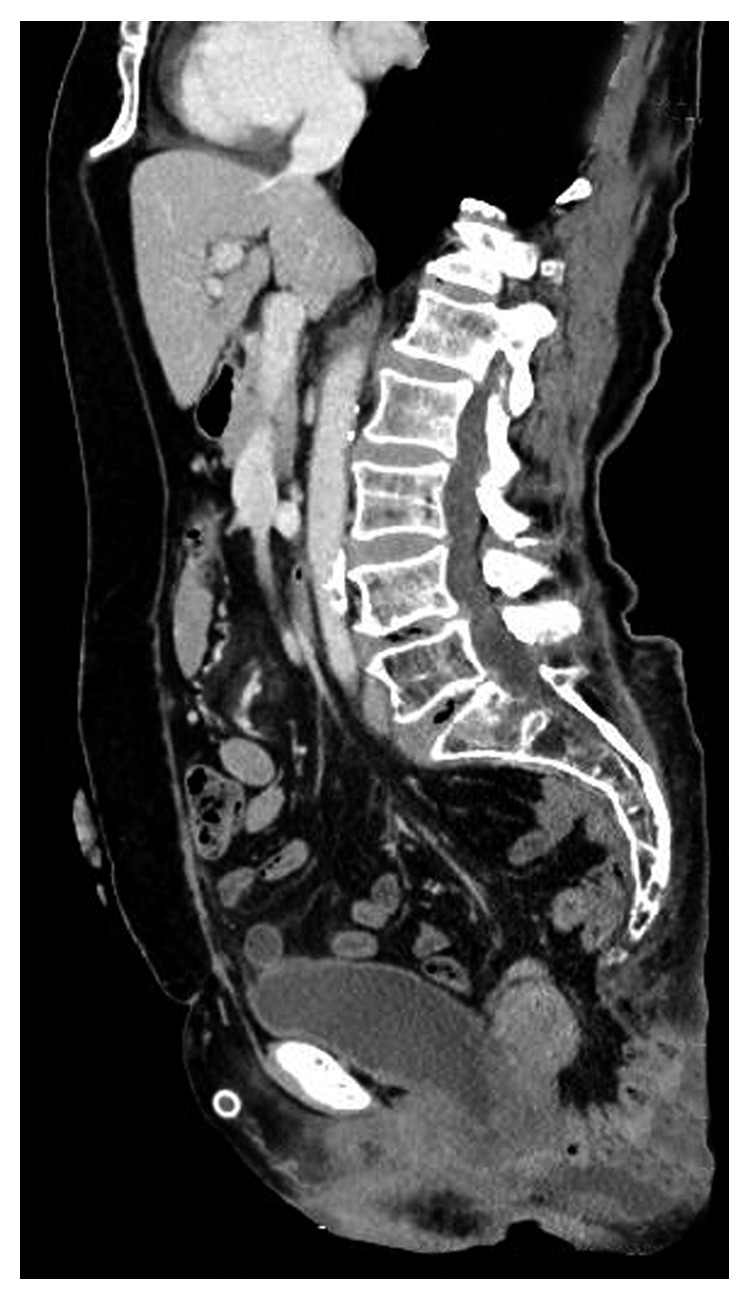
Herniated small bowel segments through the pelvis in CT scan.
